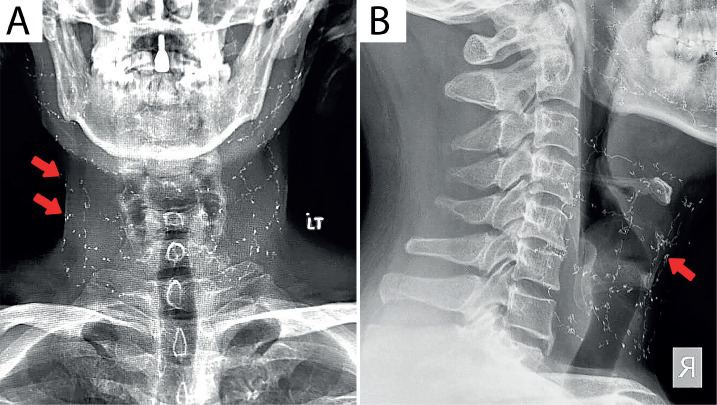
The cervical radiographs in A – AP; and B – lateral views indicate a loss of cervical lordosis, osteophytes at C5 and C6, and two layers of radiopaque strings over the anterior and bilateral sides of the cervical spine.
